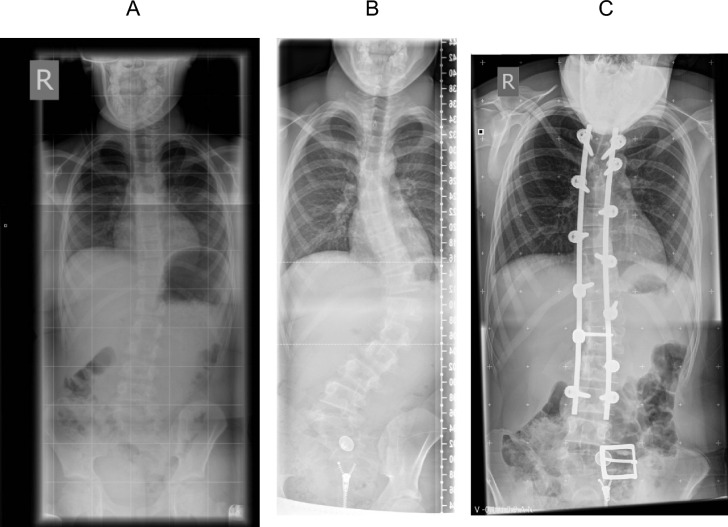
Case 1 (Case ID5); subject of this report. A PA whole spine radiograph at initial presentation demonstrates a mild scoliosis deformity. B PA whole spine radiograph demonstrating progression of the scoliosis. C Whole spine PA radiograph following surgical correction
